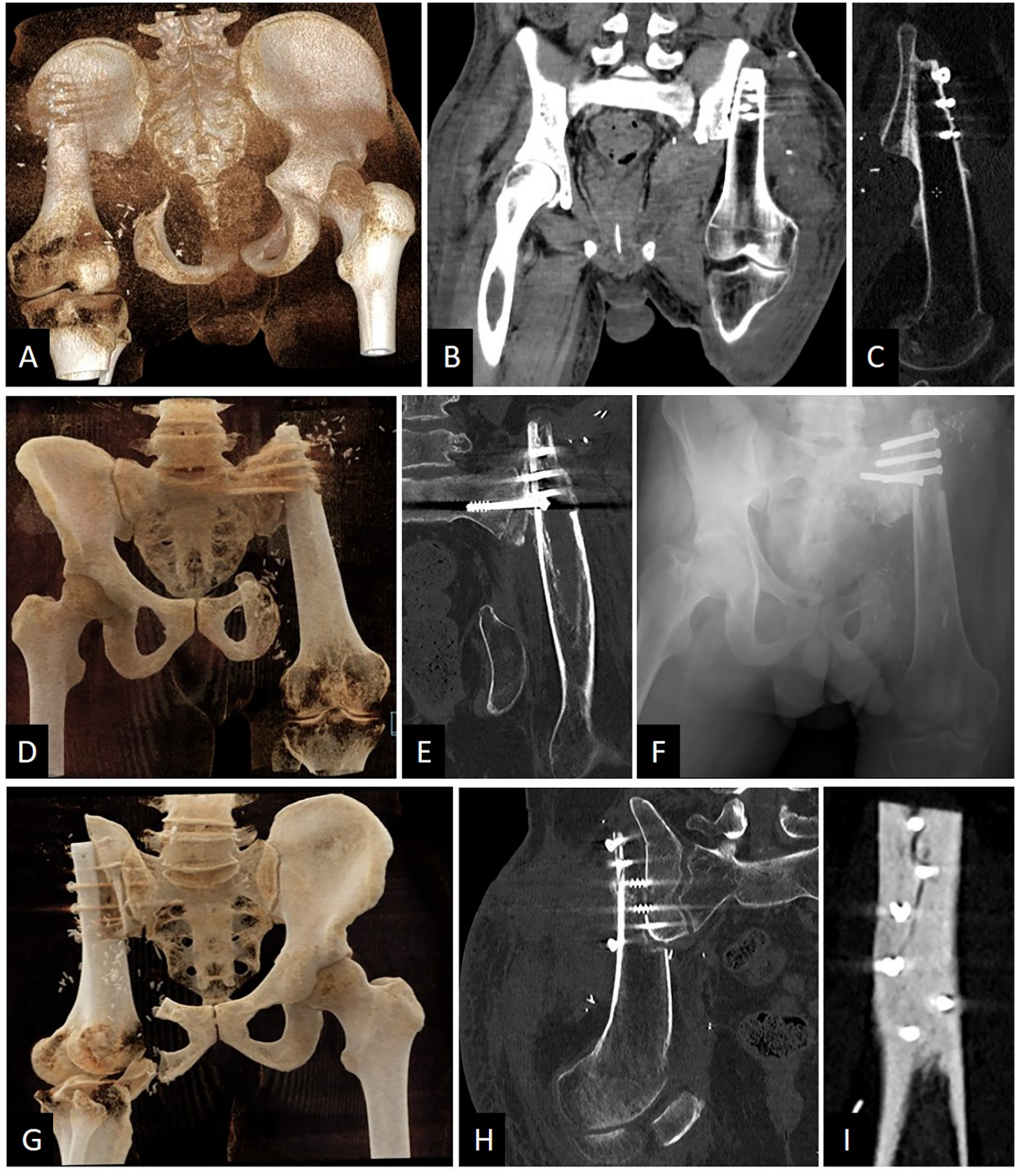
Relevant postoperative imaging of the three patients. (A) Patient #1. Posterior CT-scan 3D reconstruction showing that P3 was preserved. (B) Patient #1. CT-scan coronal view of the pelvis. Stabilization was ensured by five screws in P1. (C) Patient #1. CT-scan sagittal view of the osteosynthesis showing good consolidation ongoing after 4 months post-surgery. (D) Patient #2. Anterior CT-scan 3D reconstruction showing that P3 was preserved and P1 was resected. (E) Patient #2. CT-scan coronal view of the pelvis showing screws going in the sacral wing. (F) Patient #2. Anteroposterior x-ray view of the pelvis. Four screws were sufficient for stabilization. (G) Patient #3. Anterior CT-scan 3D reconstruction showing that P3 was partially preserved and P1 was largely resected. (H) Patient #3. CT-scan coronal view of the pelvis showing screws going in the sacral wing. (I) Patient #3. CT-scan sagittal view of the external femoral cortex fixed to the residual P1 and sacral wing. A small visible crack changed the rehabilitation postoperative care due to a risk of major fracture.
